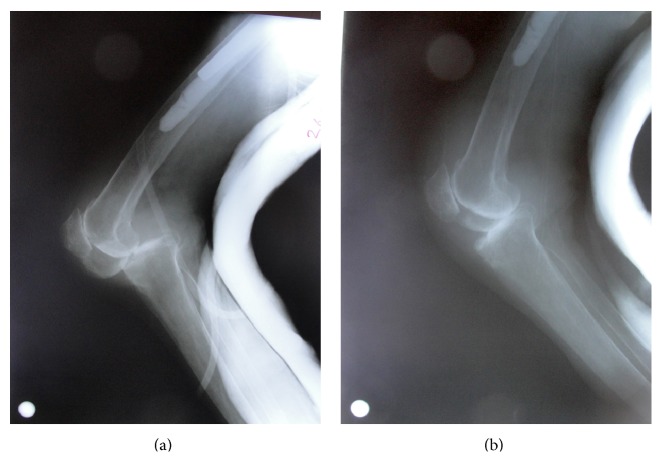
Posterior knee dislocation seen on radiography before and after reduction with underlying knee osteoarthritis.
